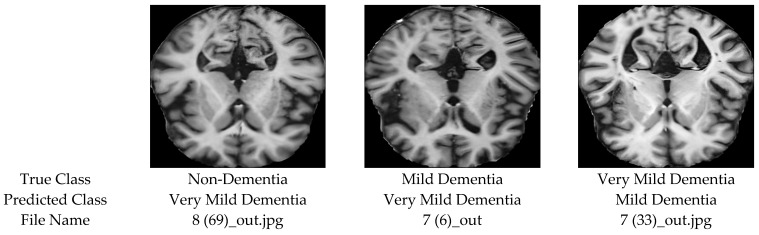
Misclassified MRI slices from the MobileNet + Swin Transformer ensemble.
